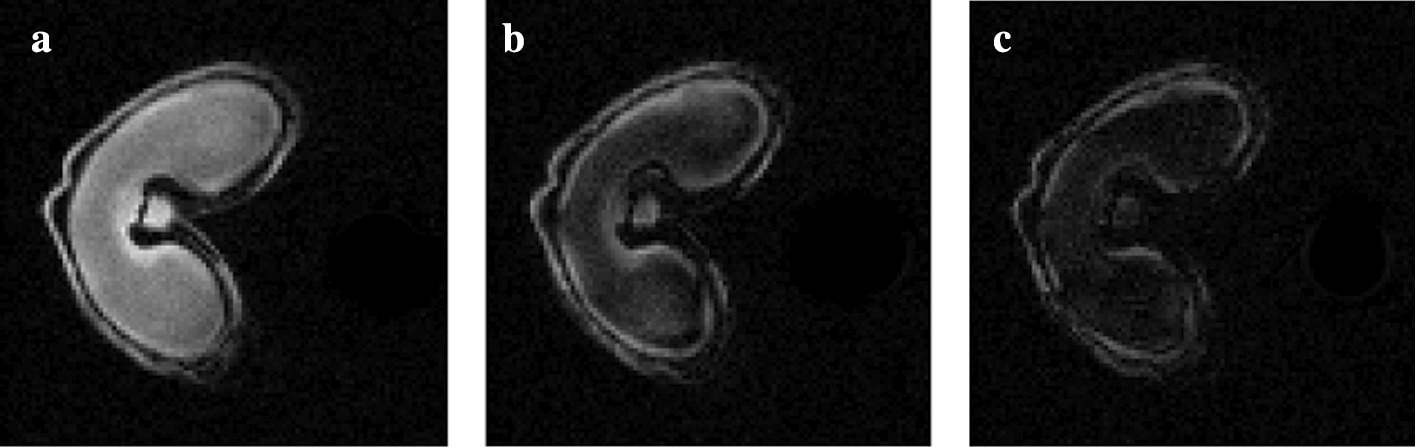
MRI images obtained at different echoes. a first echo, b second echo and c third echo. The intensity is decreasing over time following an exponential function
